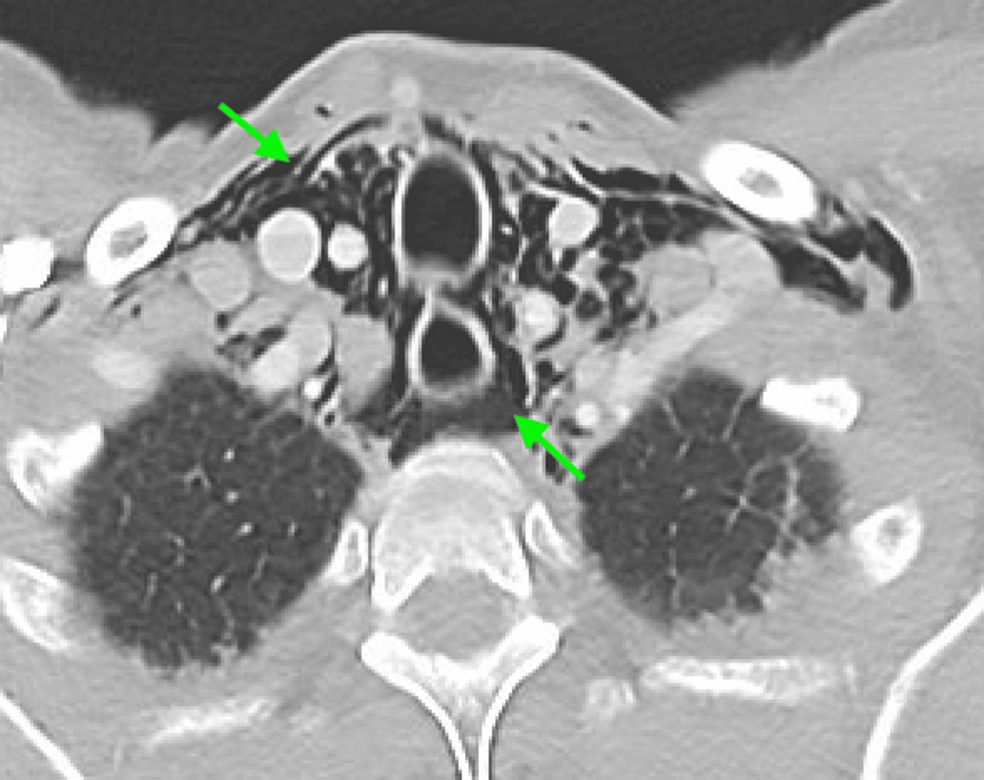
Axial slice of CT chest displaying extensive pneumomediastinum (green arrows)
